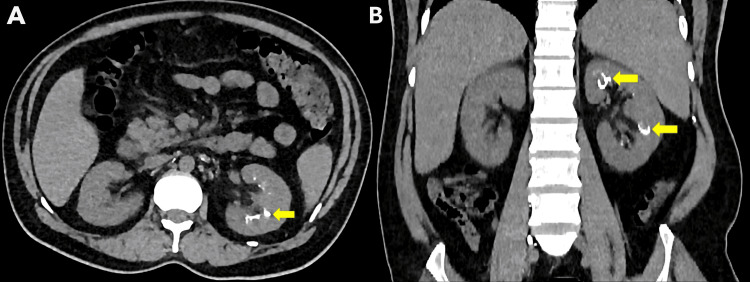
Axial (A) and coronal (B) computed tomography (CT) images obtained 12 hours after lymphangiography, demonstrating Lipiodol opacification within multiple sites of the left pelvicalyceal system (yellow arrow), confirming the presence of multiple pyelolymphatic fistulae.
